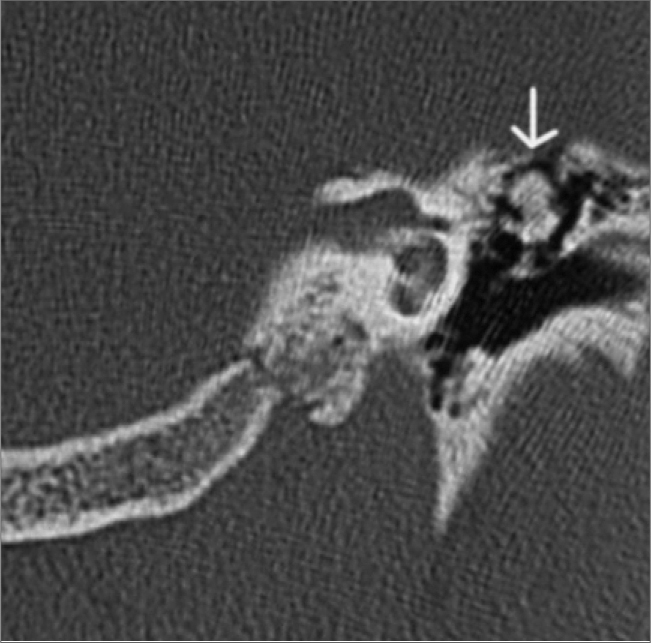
Mastoid coronal view CT scan, showing the osteoma.
